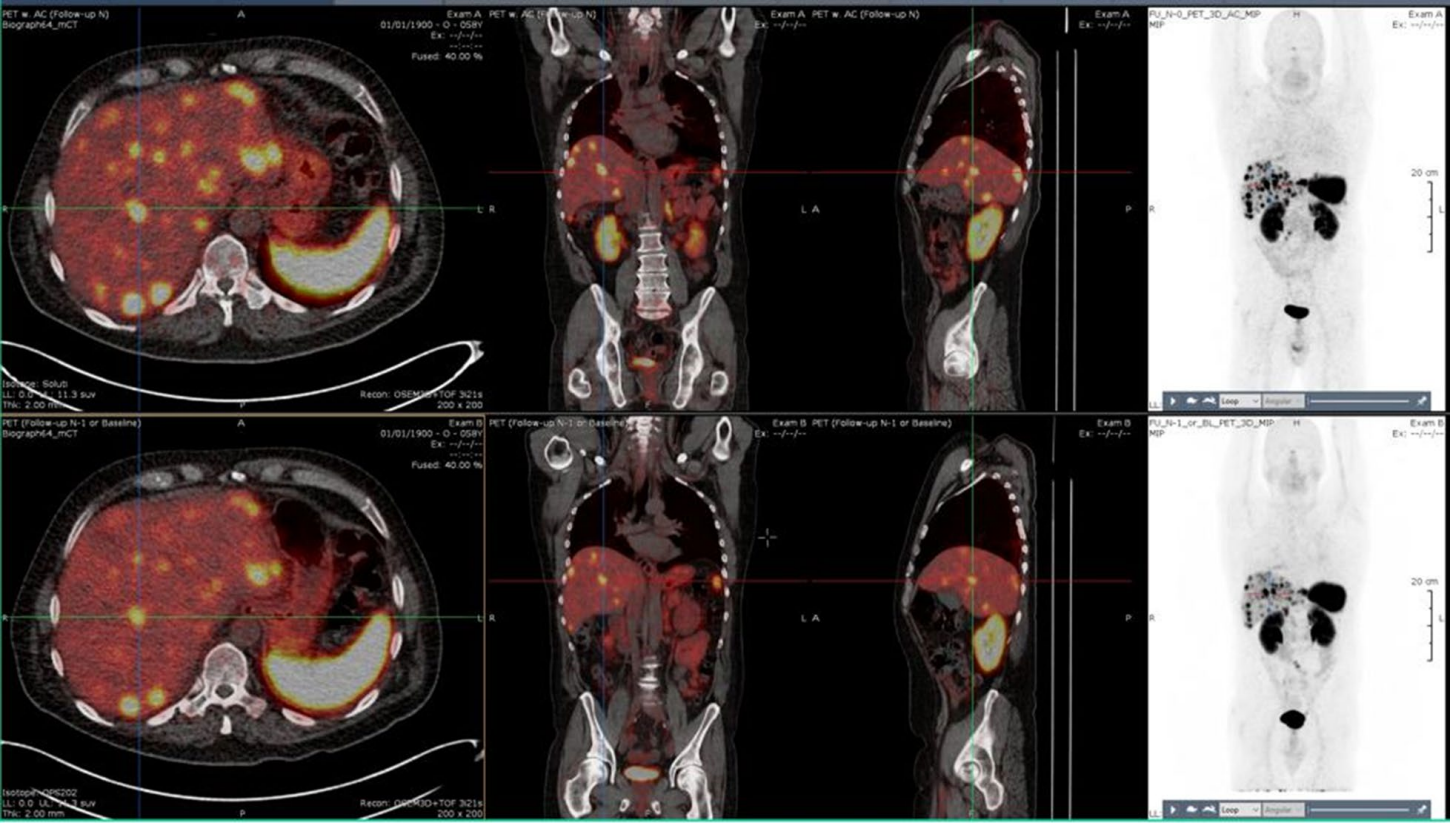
Two ^68^Ga-satoreotide trizoxetan PET/CT fused images with maximum intensity projection of the same patient acquired at two different timepoints separated by 2–3 weeks. In the top panel, the patient had received ^68^Ga-satoreotide trizoxetan at 16 µg/165 MBq. In the lower panel, the patient had received ^68^Ga-satoreotide trizoxetan at 32 µg/72 MBq. Abbreviations: CT, computed tomography; PET, positron emission tomography
